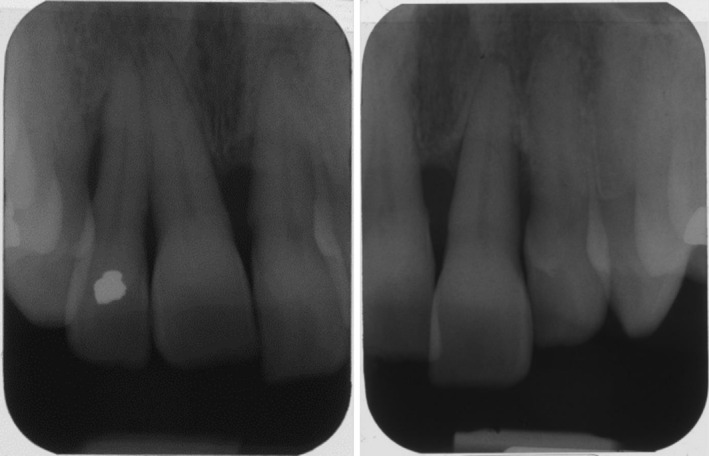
Periapical radiograph of the maxillary anterior tooth showing advanced bone loss.
